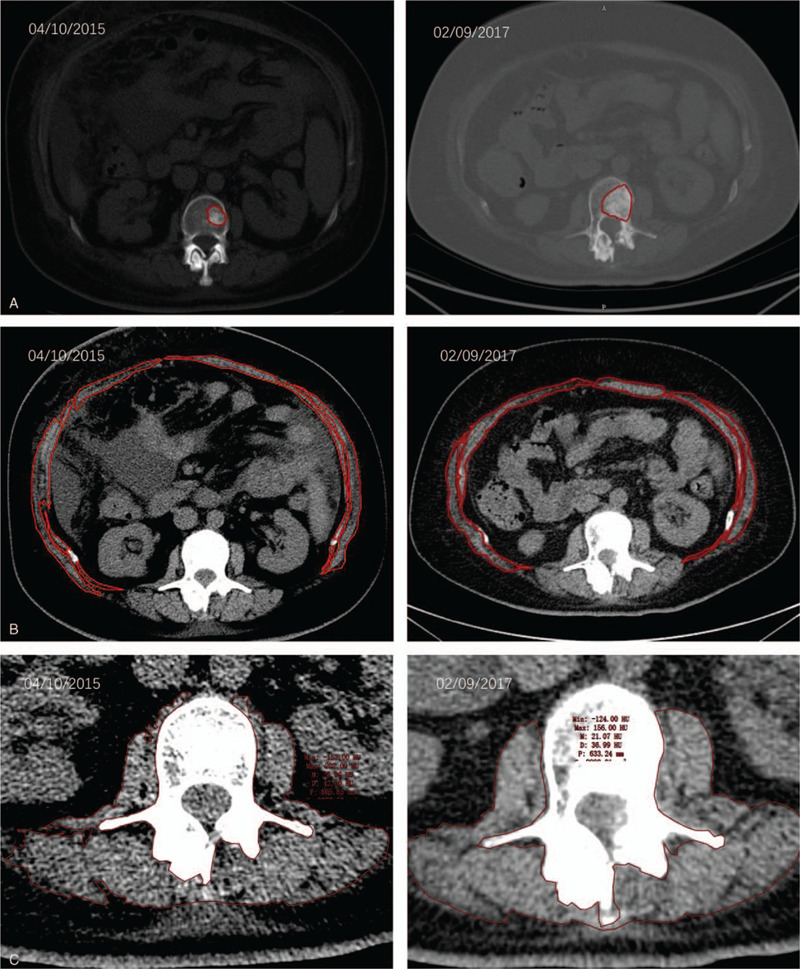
(A) TU segmentation. CT abdomen scan image at the L2 vertebral level. (B) AM segmentation. CT abdomen scan image at the L2 vertebral level. (C) PM segmentation. CT abdomen scan image at the L2 vertebral level. (D) Fat segmentation with top row of figures showing segmentation contours, and bottom row showing coloration of VF within the inside red contour and SF between the red contours. CT abdomen scan image at the L2 vertebral level.
